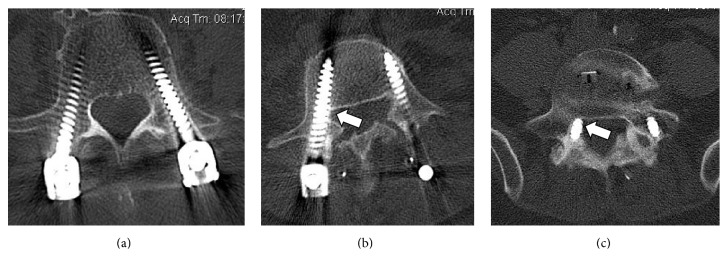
Examples of pedicle screw placement on postoperative CT imaging: (a) “correct” (no mediocaudal pedicle wall penetration), (b) “suboptimal but acceptable” (0–2 mm penetration), and (c) “misplaced” (penetration > 2 mm).
